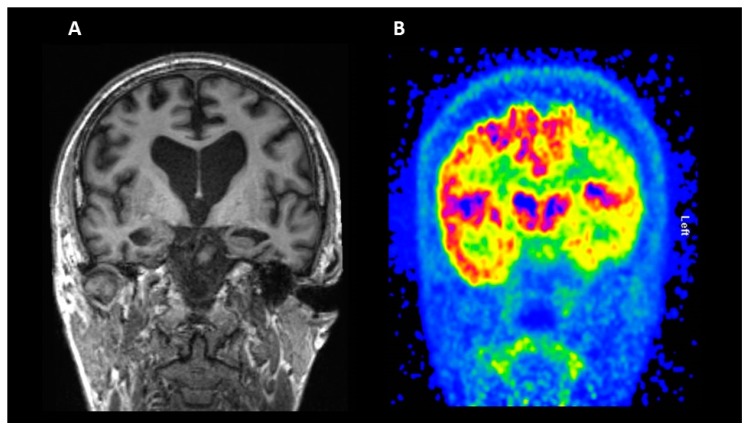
Imaging biomarkers of neurodegeneration. Coronal structural MRI section (panel A) and 18F-fluorodeoxyglucose (FDG) PET (panel B) from a patient with Alzheimer’s disease (AD).
